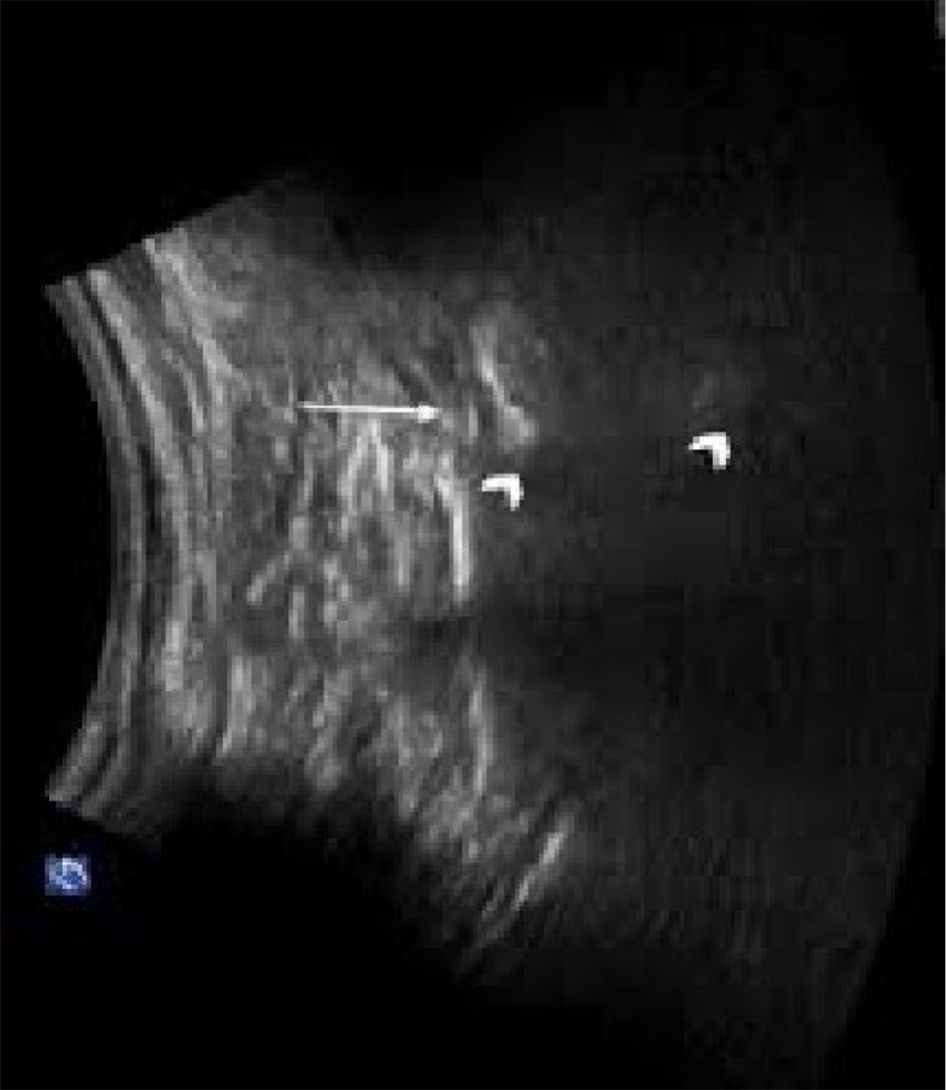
The S1 transforaminal view in ultrasound
